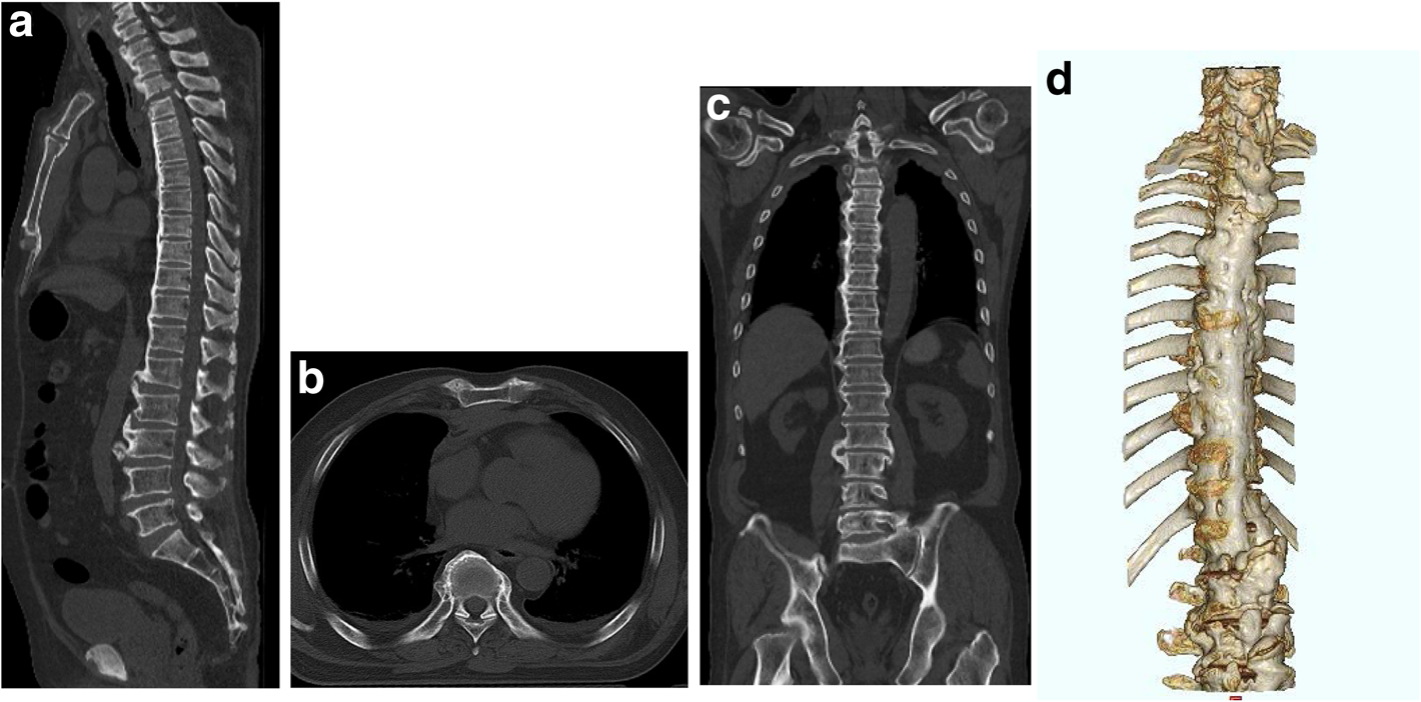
CT images of a patient with DISH. Reconstructed CT of sagittal (a), axial (b), coronal (c) and 3D (d) views in a patient with DISH
